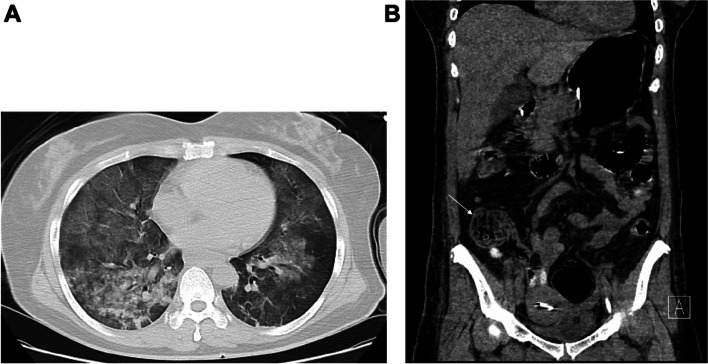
Computed tomography images. A Pulmonary CT revealed exudation and edema on day 1. B Abdominal CT revealed the edema of ascending colon on day 6
